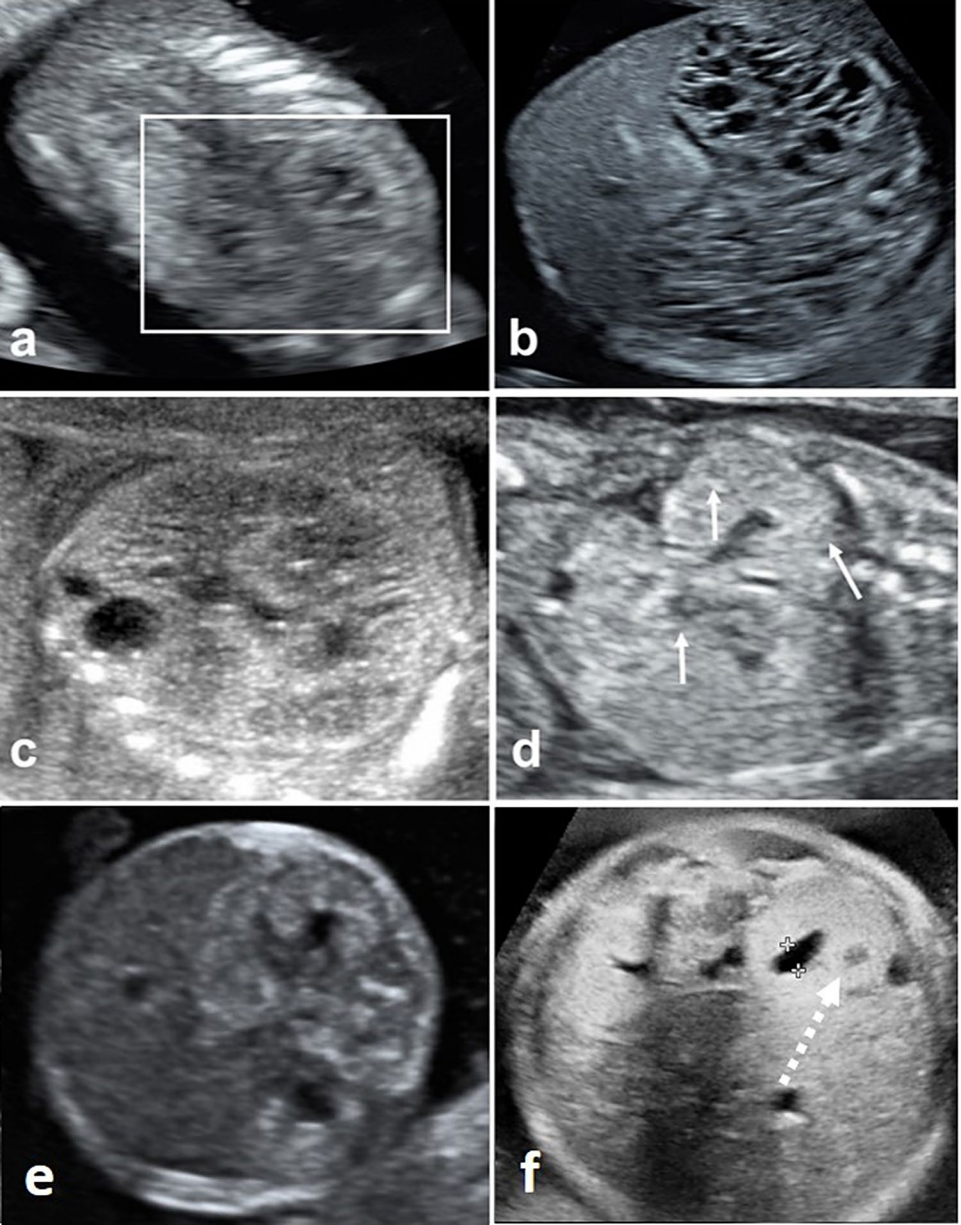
Prenatal ultrasound in fetuses with Meckel-Gruber syndrome (*MKS*, a–c) and Simpson–Golabi–Behmel syndrome (*SGBS*, d-f). a Shows a fetus with *MKS* at 12+0 weeks’: corticomedullary differentiation (*CMD*) appears premature or “too advanced” for the gestational age (hyperechogenic cortex, hypoechogenic medulla; white box); b shows the same fetus at 14+5 weeks’: both kidneys (axial view) present with multiple cysts of different size both in cortex and medulla (with accentuated occurrence in medulla, causing for the “mottled appearance”); c shows a different fetus at 19+4 weeks’ with enlarged kidneys presenting with absent *CMD* and cysts of various size interspersing the entire parenchyma; d–e show a fetus with *SGBS* in 17+4 weeks’, presenting with mildly enlarged, hyperechogenic kidneys showing absent corticomedullary differentiation (*CMD*, coronary and axial view) as well as small cysts (white arrows); e shows another fetus with SGBS at 31+3 weeks’, presenting with enlargend and hyperehogenic kidneys with absent *CMD* and small cysts (white arrow) as well as mild hydronephrosis (axial view, dotted white arrow)
